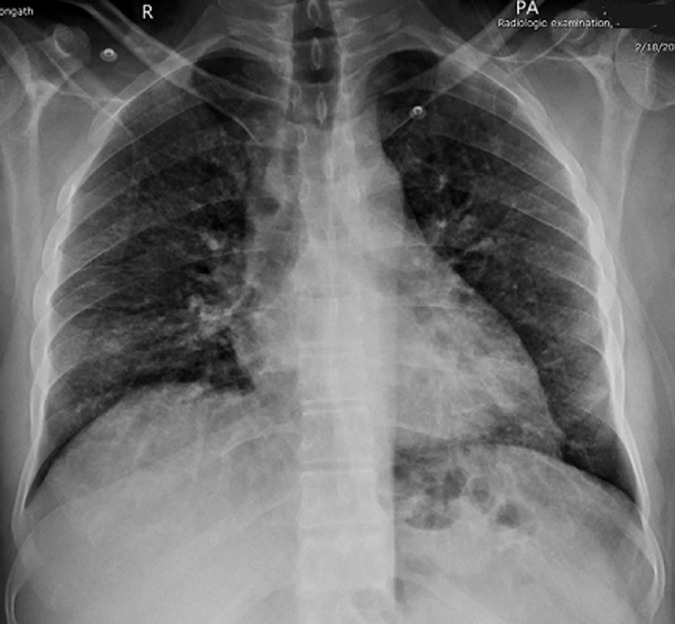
initial chest X-ray taken on 18.2.21 showed bilateral ground glass opacities (GGO) predominantly in the mid and lower zones; no mediastinal abnormality is noted and both costophrenic angles are clear
